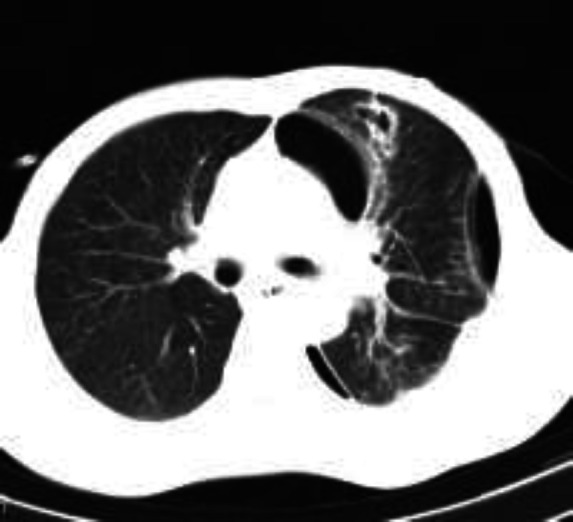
Lung HRCT after laparatomy and chest tube drainage revealing left sided hydropneumothorax. Arrow pointing at a peripheral cavity in the left lung parenchyma
